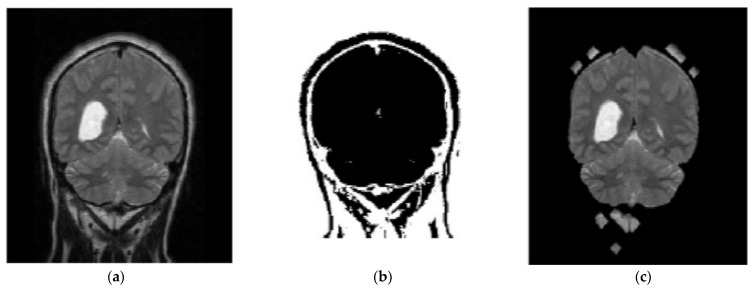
Brain MRI images: (a) normal; (b) binary; (c) after a morphological operation.
